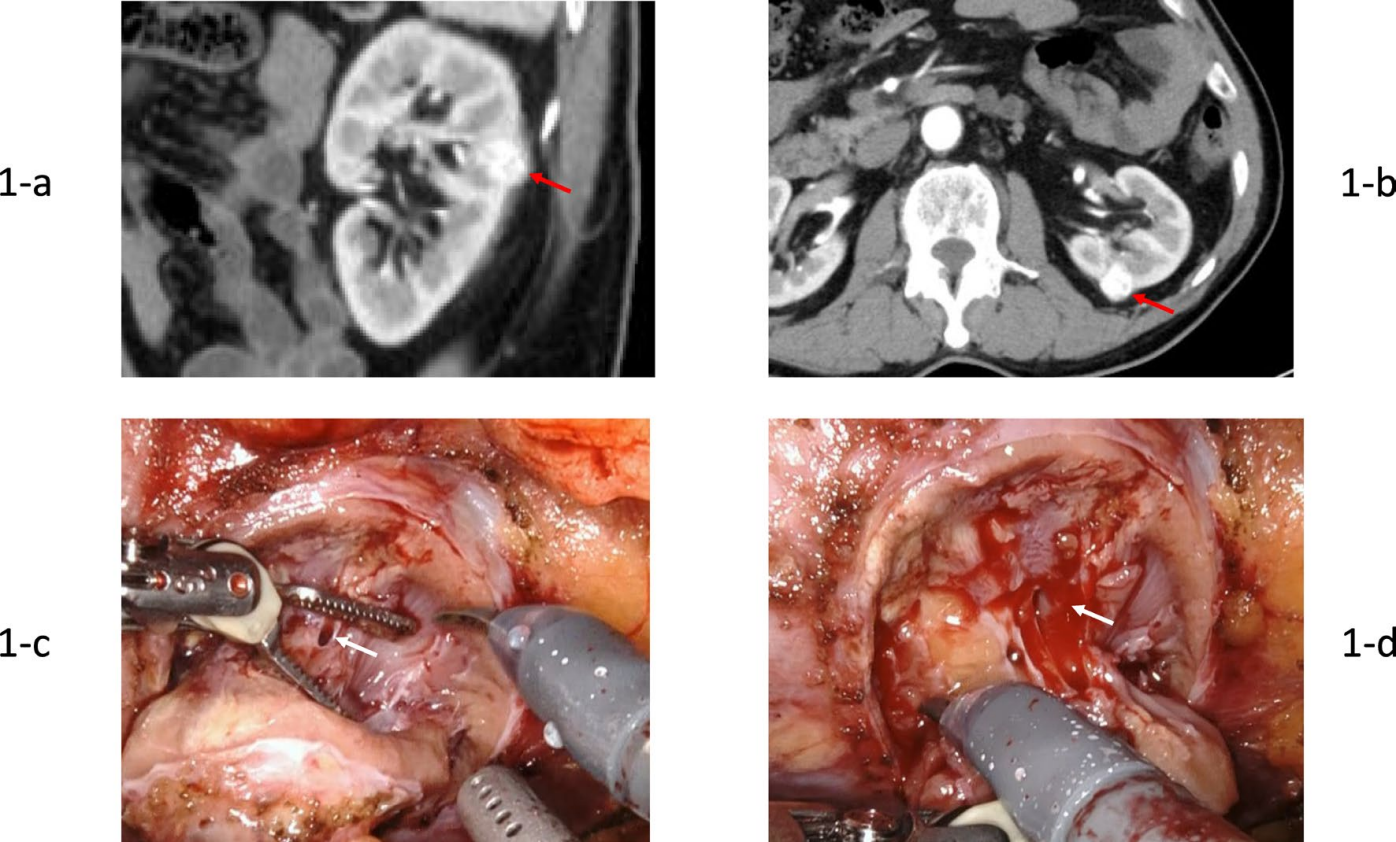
(a and b) Abdominal contrast‐enhanced CT. An 18 × 14 mm tumor was observed on the dorsal aspect of the left kidney, with marked contrast enhancement in the arterial phase (RENAL nephrometry score 6p). (c and d) Intraoperative findings. A small vein (1–2 mm) was opened approximately 4 min after initiating partial nephrectomy. Arrow indicates the opened vein.
